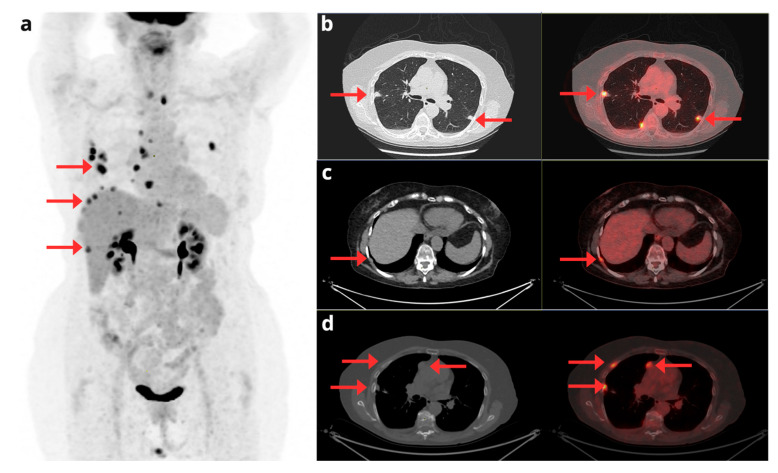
A 75-year-old woman with a history of stage IB FIGO clear cell EC, treated in April 2022 with total hysterectomy, bilateral salpingo-oophorectomy, pelvic and aortocaval lymphadenectomy, and omentectomy, followed by adjuvant chemoradiotherapy and brachytherapy. Due to the appearance of pulmonary nodules on a follow-up CT scan, a [^18^F]F-FDG PET/CT was requested. The study revealed distant disease recurrence, with the maximum intensity projection PET image (a) demonstrating multiple pulmonary nodules, pleural implants, and bone involvement (red arrows). Axial CT and PET/CT slices (b–d) confirmed foci of pathological hypermetabolism corresponding to bilateral pulmonary nodules (b), an implant in the right costal pleura (c), a mediastinal lymphadenopathy in a prevascular location, a subpleural pulmonary nodule in the right lower lobe, and the fourth right anterior costal arch (d).
